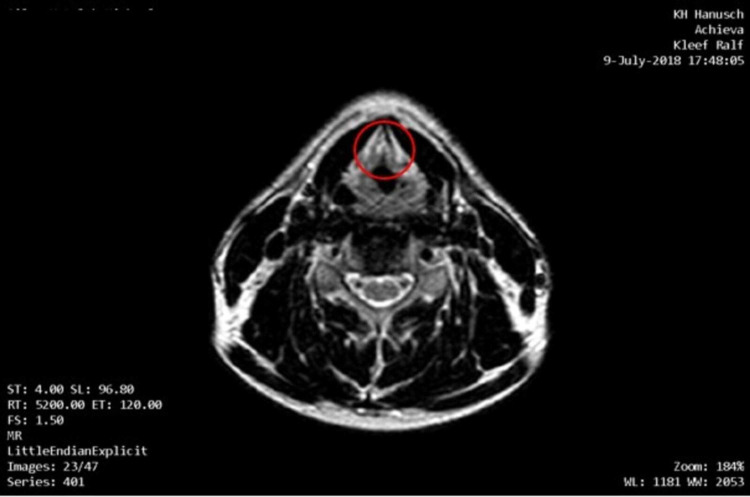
MRI with contrast agent from July 2018. After therapy regular configuration of vocal cords and commissura anterior, no detectable tumor tissue, no signs of edema, showing complete remission.
